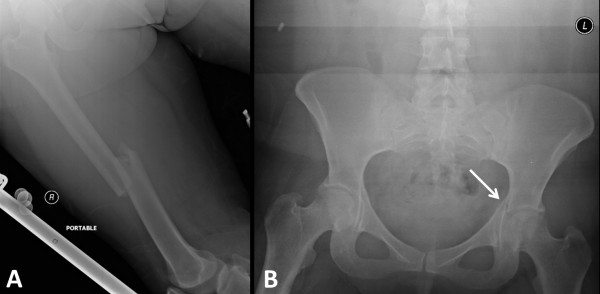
Initial radiographic workup of a 28-year-old woman involved in a high-speed motor vehicle accident, sustaining a right femoral shaft fracture (A) and a contralateral nondisplaced associated transverse/posterior wall acetabular fracture (arrow in B).
